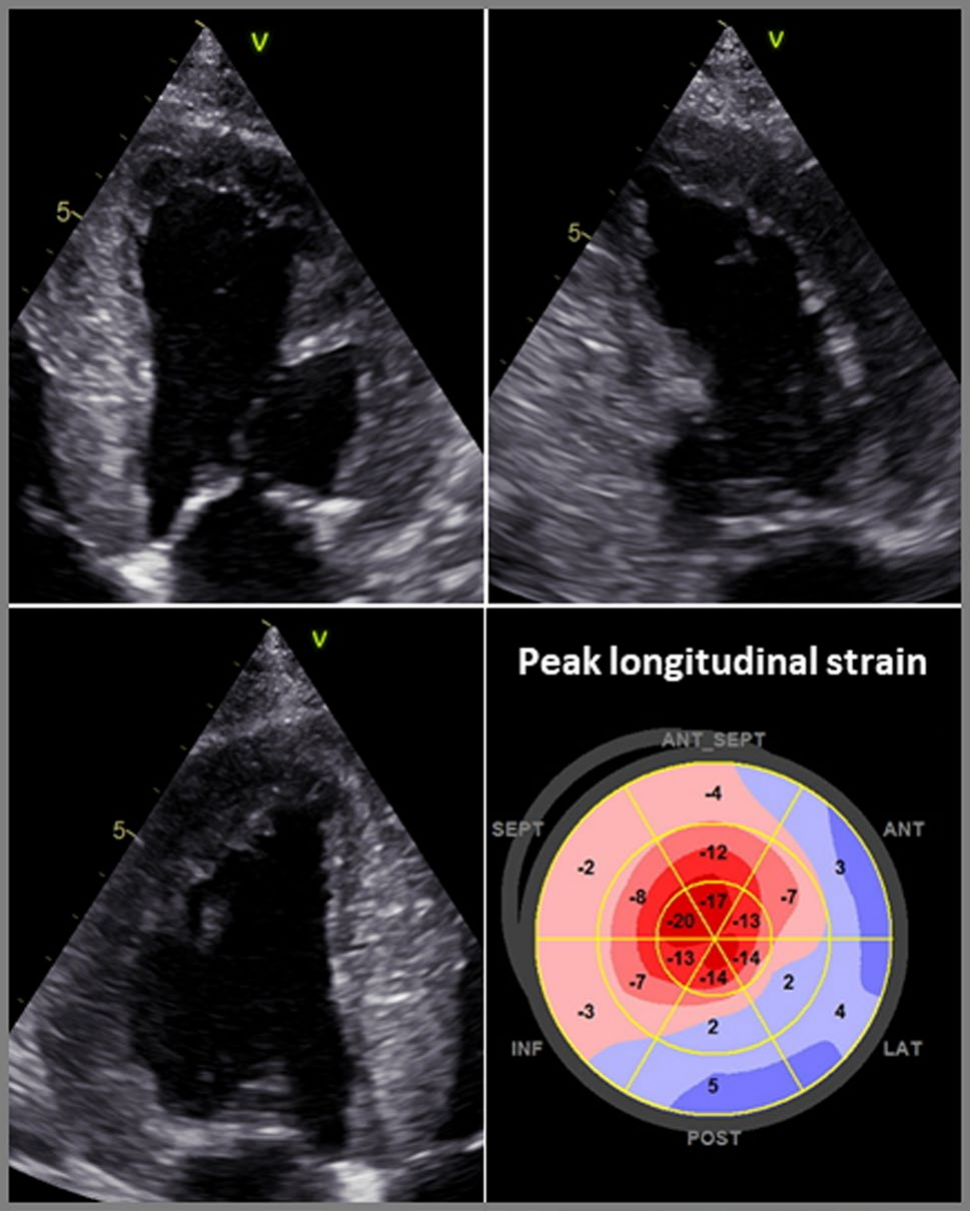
Transthoracic echocardiography with measurement of longitudinal strain and detection of a characteristic “apical sparing” phenomenon in a patient with cardiac amyloidosis
